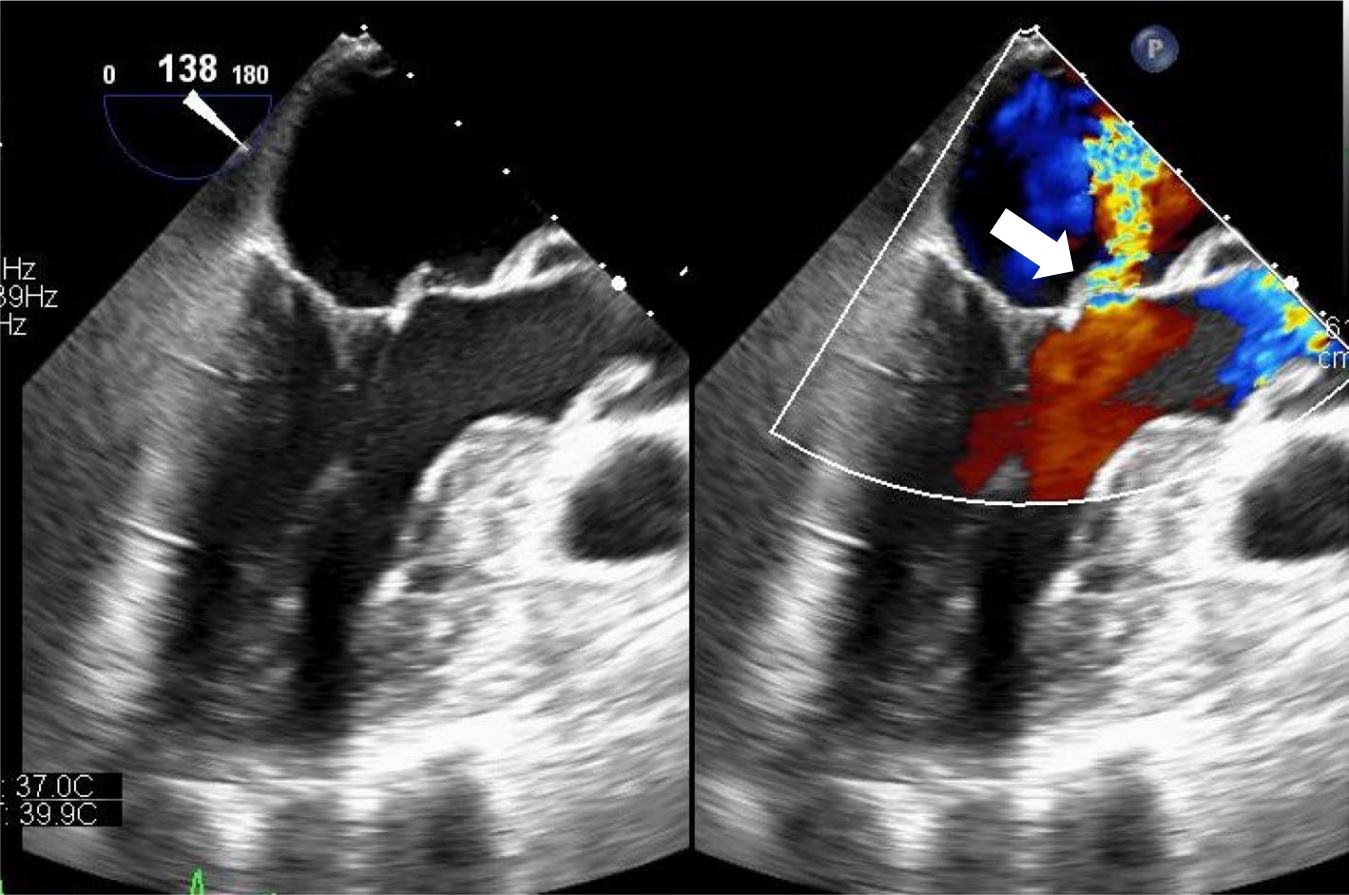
Transesophageal echocardiogram findings of the mitral valve. Perforation of the anterior mitral leaflet caused due to infection (right) (arrow)
